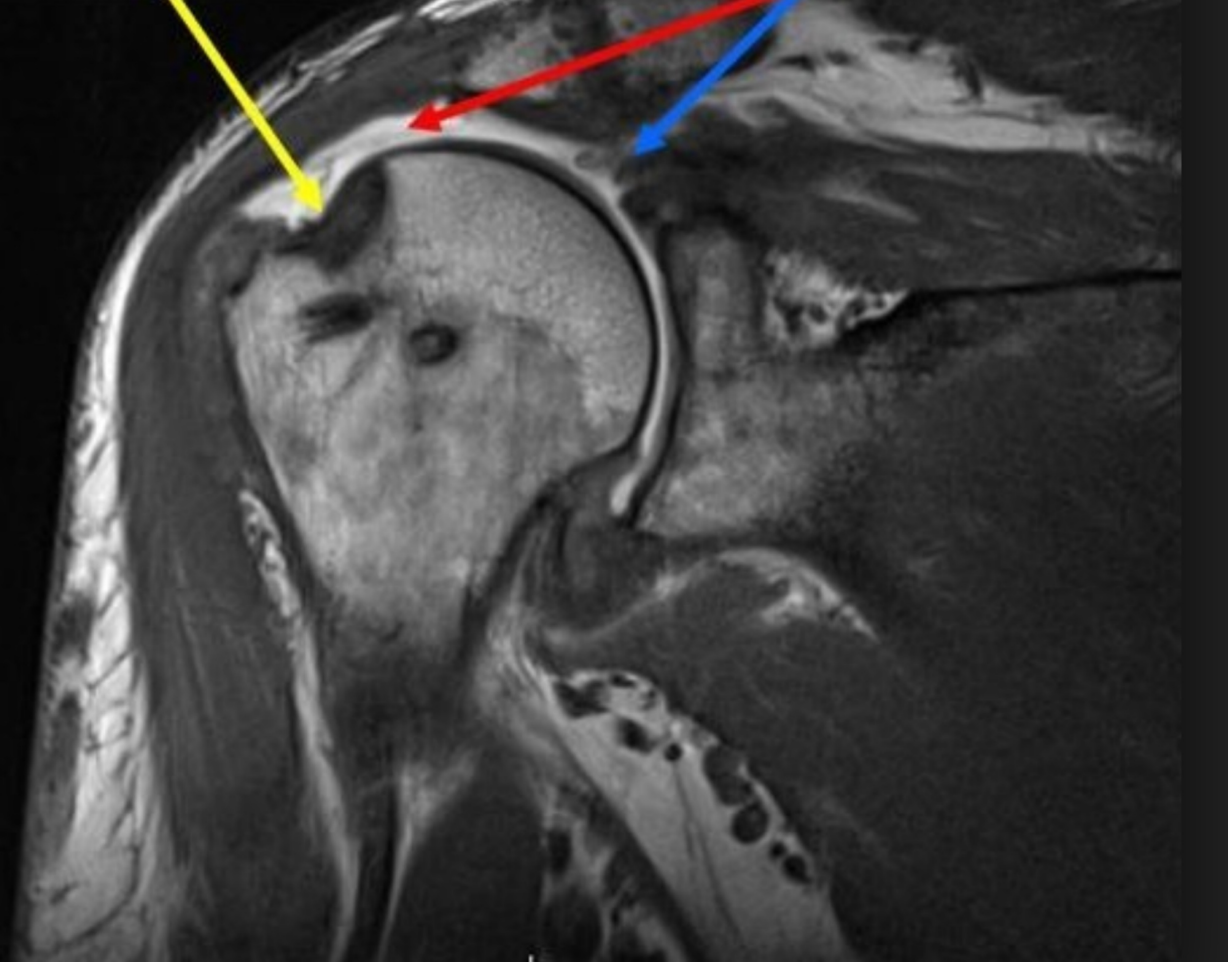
Rotator cuff tear - MRI Magnetic resonance imaging (MRI) showing a rotator cuff tear. Yellow - insertion site of supraspinatus; Red - space where the supraspinatus is present; Blue - retracted tendon of the supraspinatus muscle.
